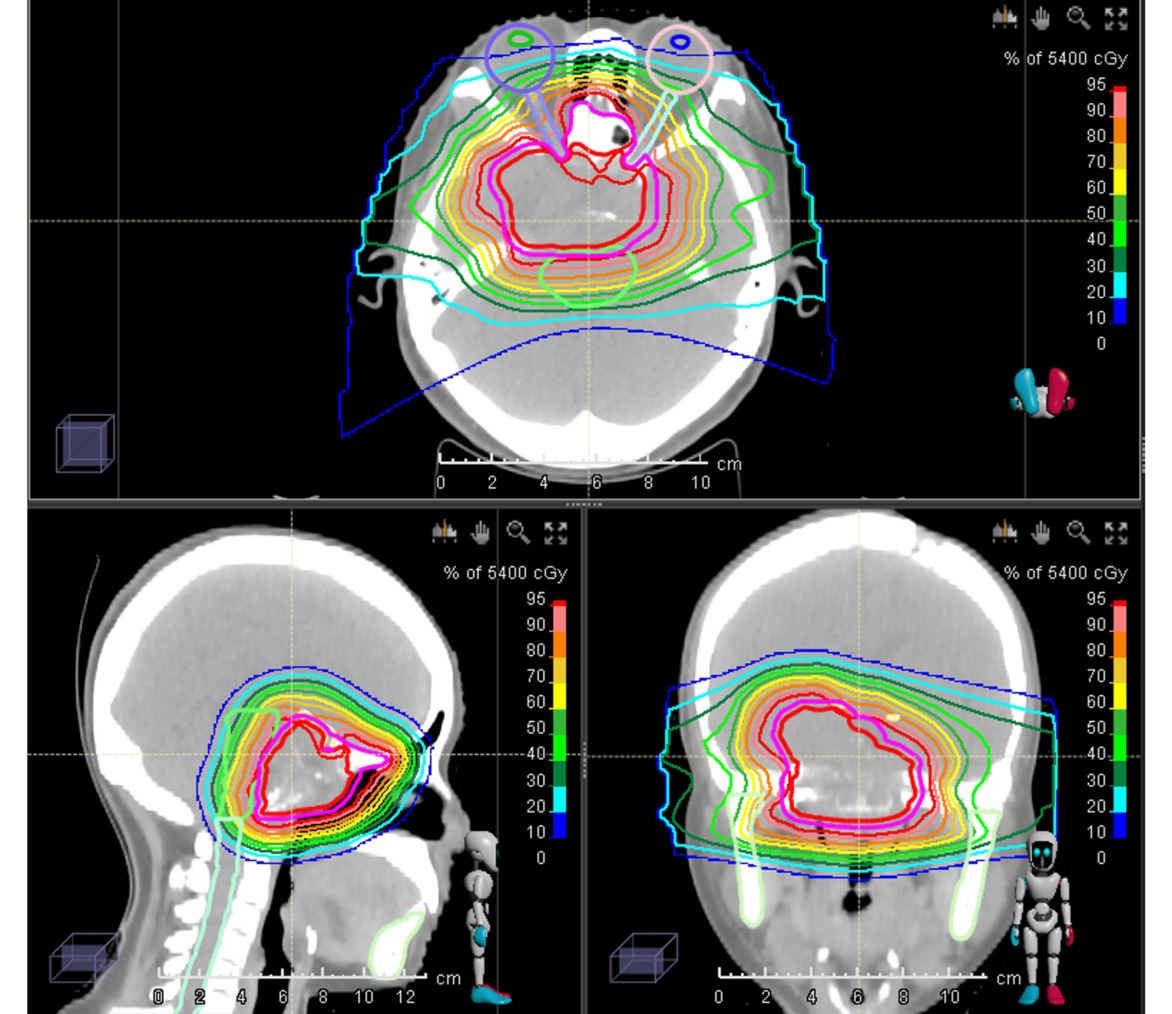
Target Volume Delineation and Planning Image Fusion: Planning CT and planning MRI scans were co-registered for target definition. GTV: The solid tumor, located in the sellar region, was delineated as the GTV based on fused imaging. CTV: The CTV was generated by adding a 2 mm isotropic margin around the GTV. Treatment Plan Prescription: The prescribed dose to the CTV was 54 Gy in 30 fractions (CTV: 54 Gy/30 f). Dose Constraint: The maximum dose to the optic chiasm was constrained to <52 Gy. GTV, gross tumor volume; CTV, clinical target volume; CT, computed tomography; MRI, magnetic resonance imaging
